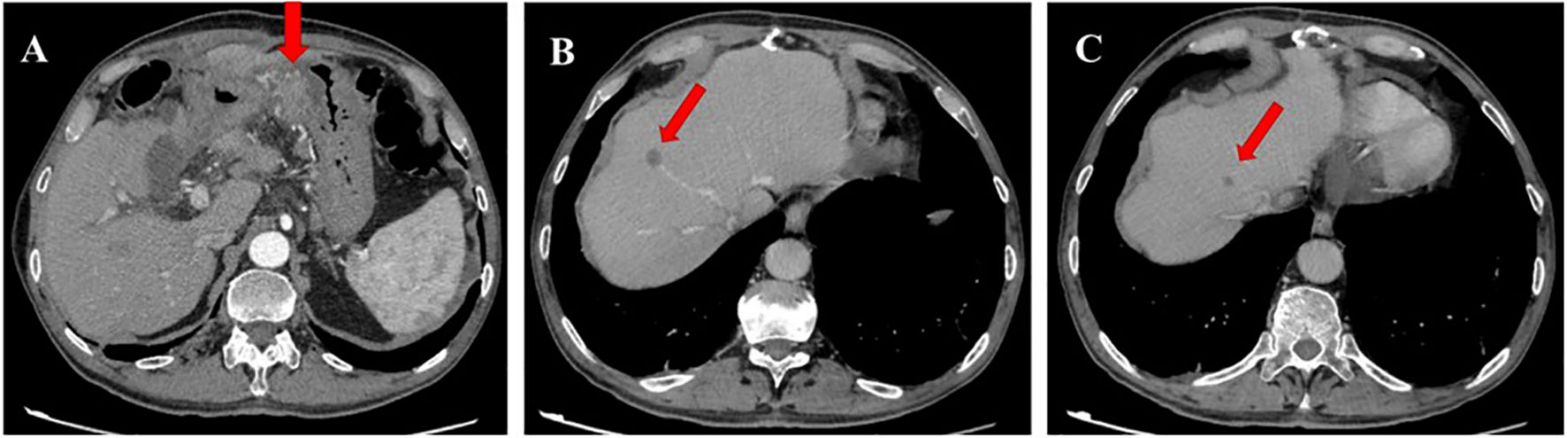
Contrast-enhanced computed tomography (CT) scan image in January 2021. (A) CT scan shows progression of infiltrative changes in the stomach. (B, C) CT scan shows new metastatic foces in the liver.
